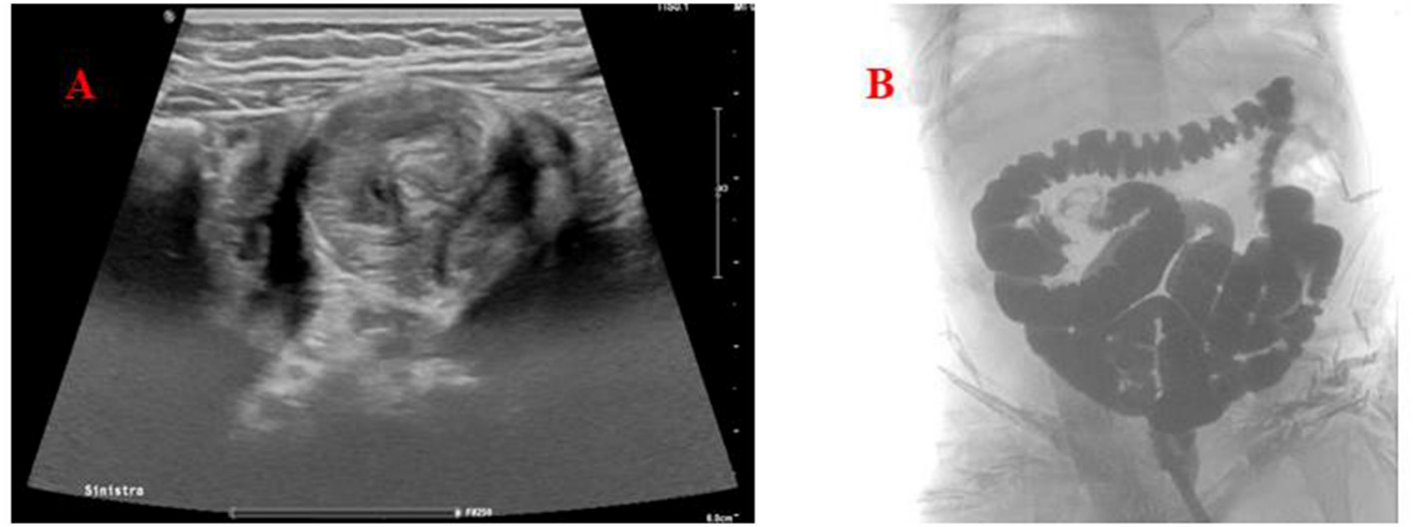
(A) Abdomen ultrasound. The target (donut) sign found in the left lower quadrant, confirming the diagnosis of intussusception. (B) Hydrostatic reduction of intussusception using Gastrografin® contrast solution showing the successful reduction of the ileocolic invagination.
